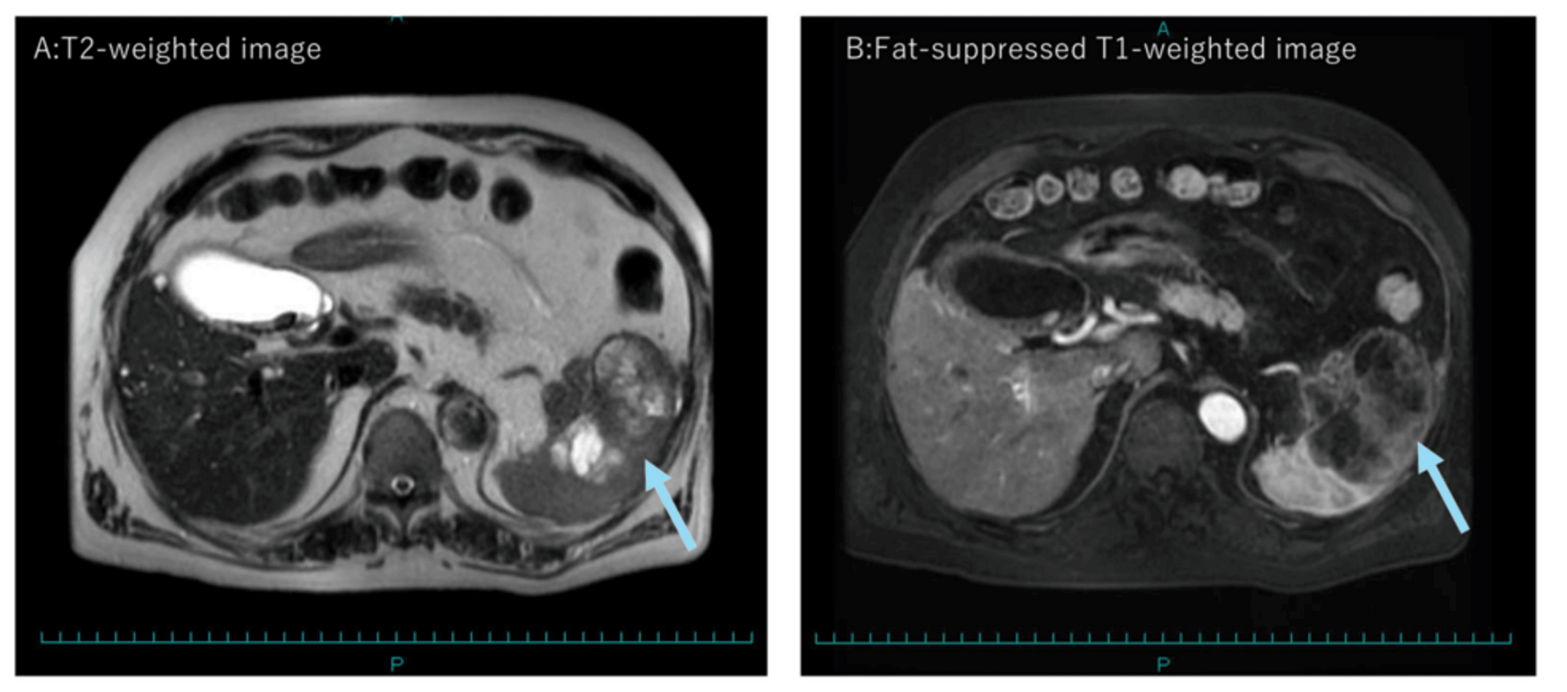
Magnetic resonance imaging of the spleen (A) The T2-weighted image shows a large mass with heterogeneous high signal intensity (arrow). (B) The fat-suppressed, T1-weighted dynamic contrast-enhanced image reveals irregular enhancement within the tumor (arrow).
